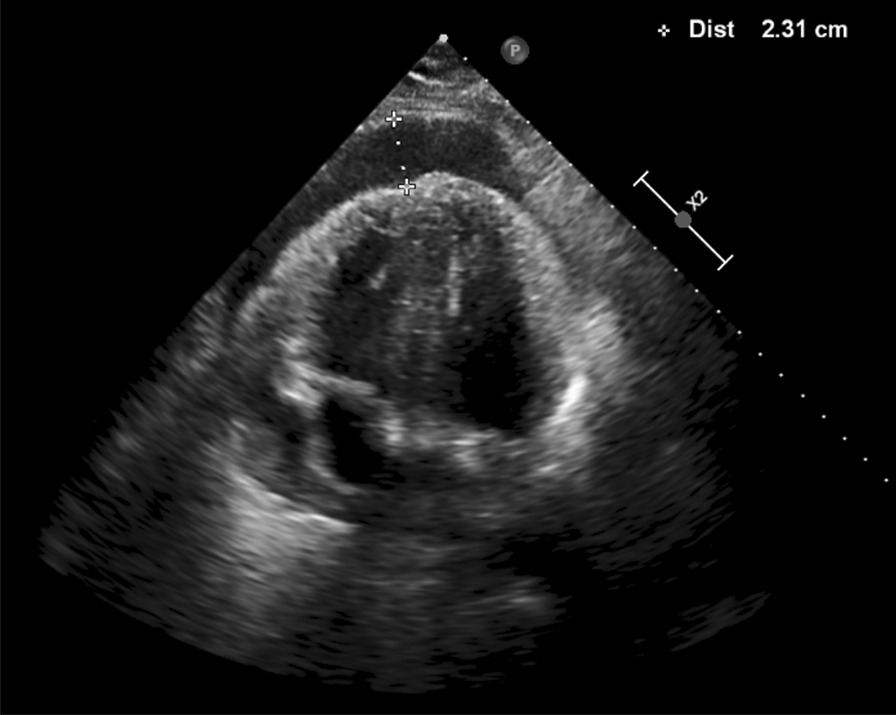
Transthoracic echocardiogram showing pericardial effusion (calipers)
